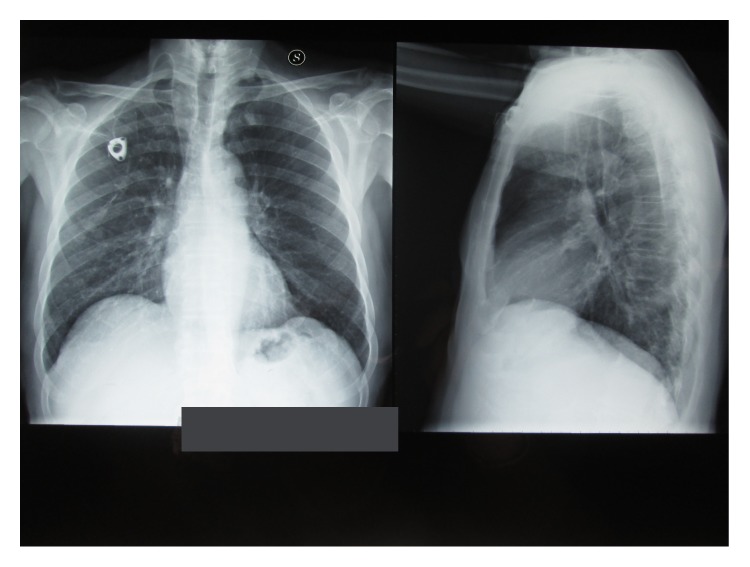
Chest X-ray after lipid formulation of amphotericin B therapy.
